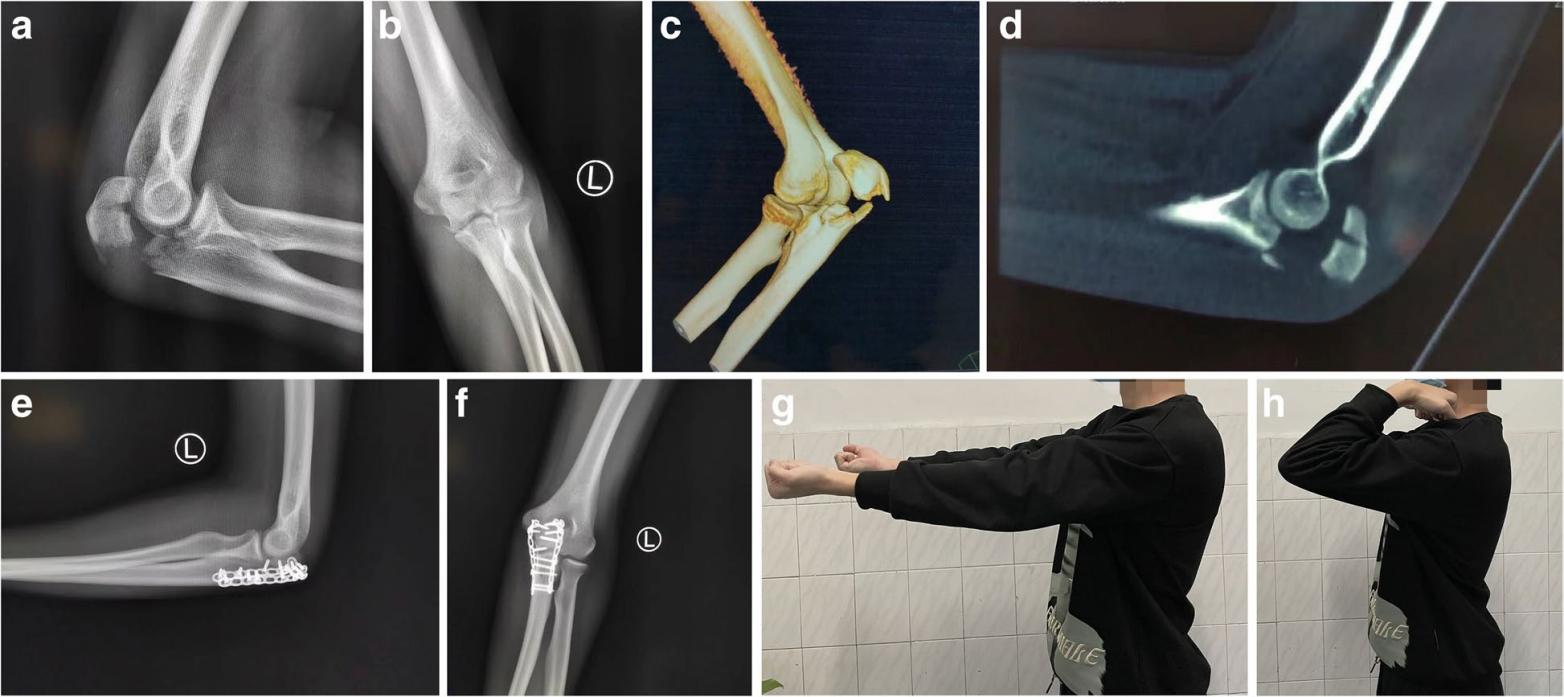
A 26-year-old man underwent surgery with double mini-locking plates. a b c d Anteroposterior and lateral X-ray film and three-dimensional CT of the elbow joint before the operation; e f postoperative anteroposterior and lateral X-ray film of the elbow joint; g h image of the patient’s elbow joint function after surgery
